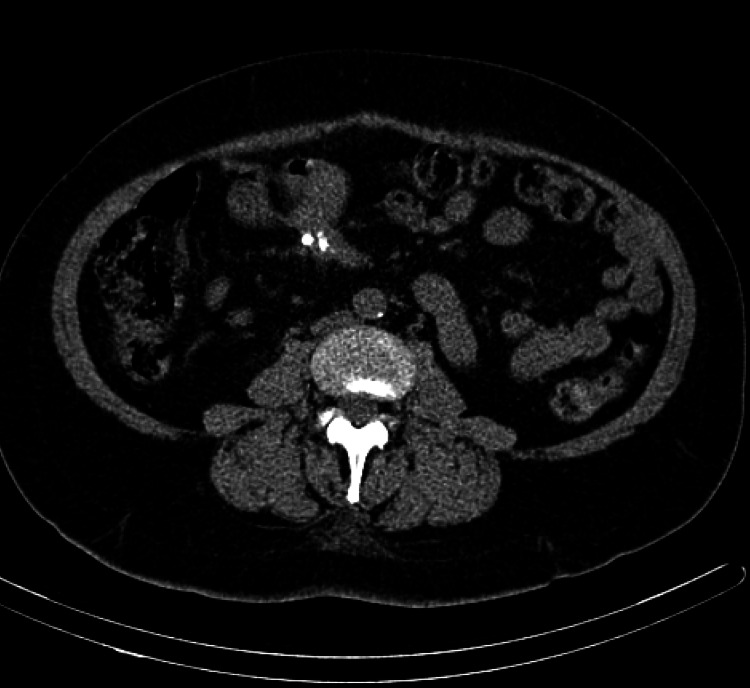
Axial CT without intravenous or enteric contrast demonstrating focal small bowel wall thickening with adjacent partially calcified mesenteric soft tissue lesion (this represents a mesenteric met with desmoplastic reaction from tumor secretion of serotonin) CT, computerized tomography.
